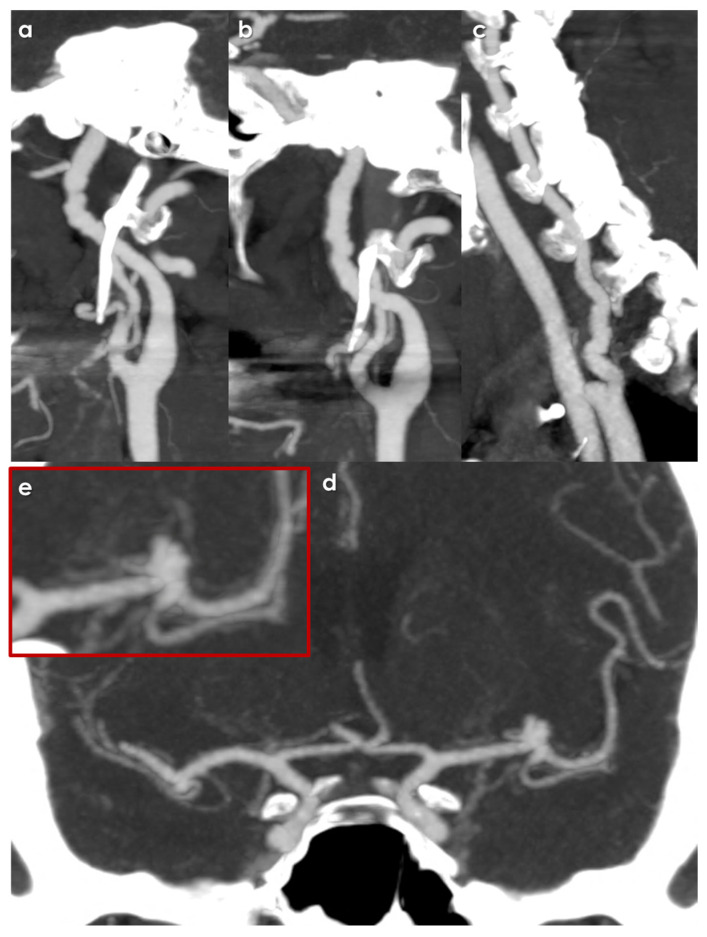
CT Angiography of the cervical and intracranial arteries performed as screening for FMD in a patient who underwent a spontaneous coronary artery dissection (renal and visceral arteries were also explored without abnormalities). All images are Multiplanar Reconstructed and processed with Maximum Intensity Projection protocol (MIP/MPR). Panel (a) shows the right extracranial ICA with the multifocal pattern (string of beads), as for left ICA (panel (b)) and left V1 VA (panel (c)). In panel (d) and (e, magnified view), a left MCA bifurcation aneurysm is evident (well detailed using catheter angiography, showed in Figure 2).
